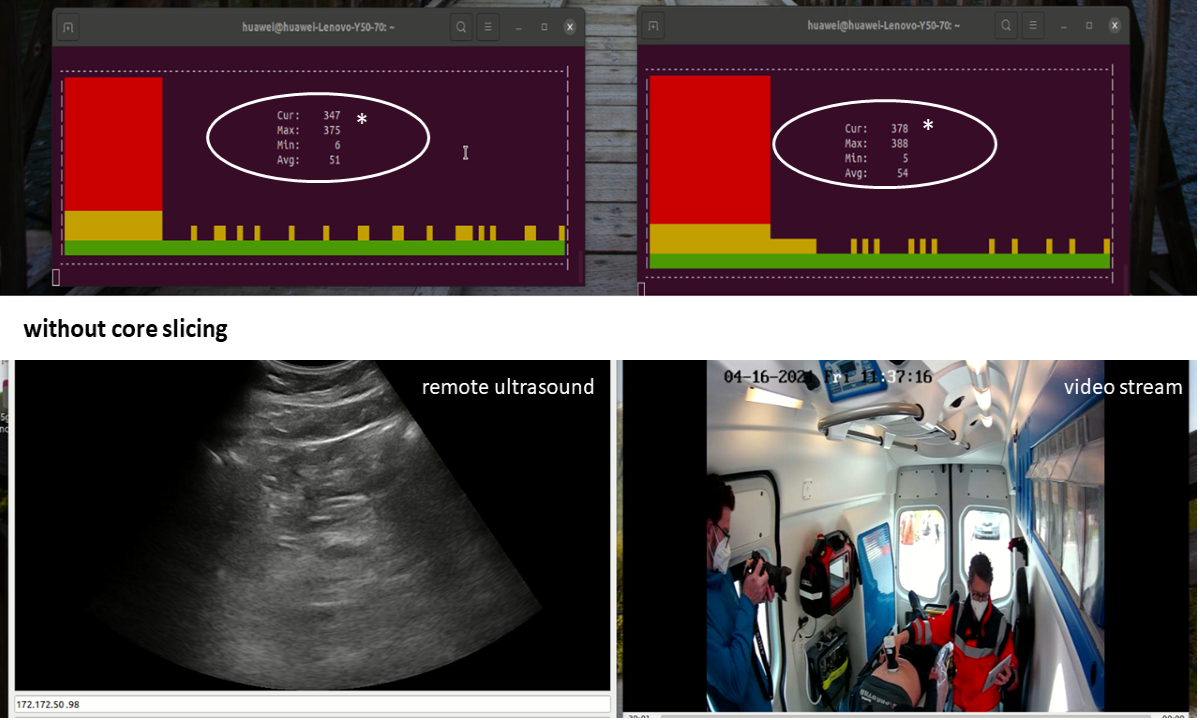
Additional uplink traffic (end-to-end round trip latency *) without core slicing. Ultrasound application (left) and video streaming (right).
